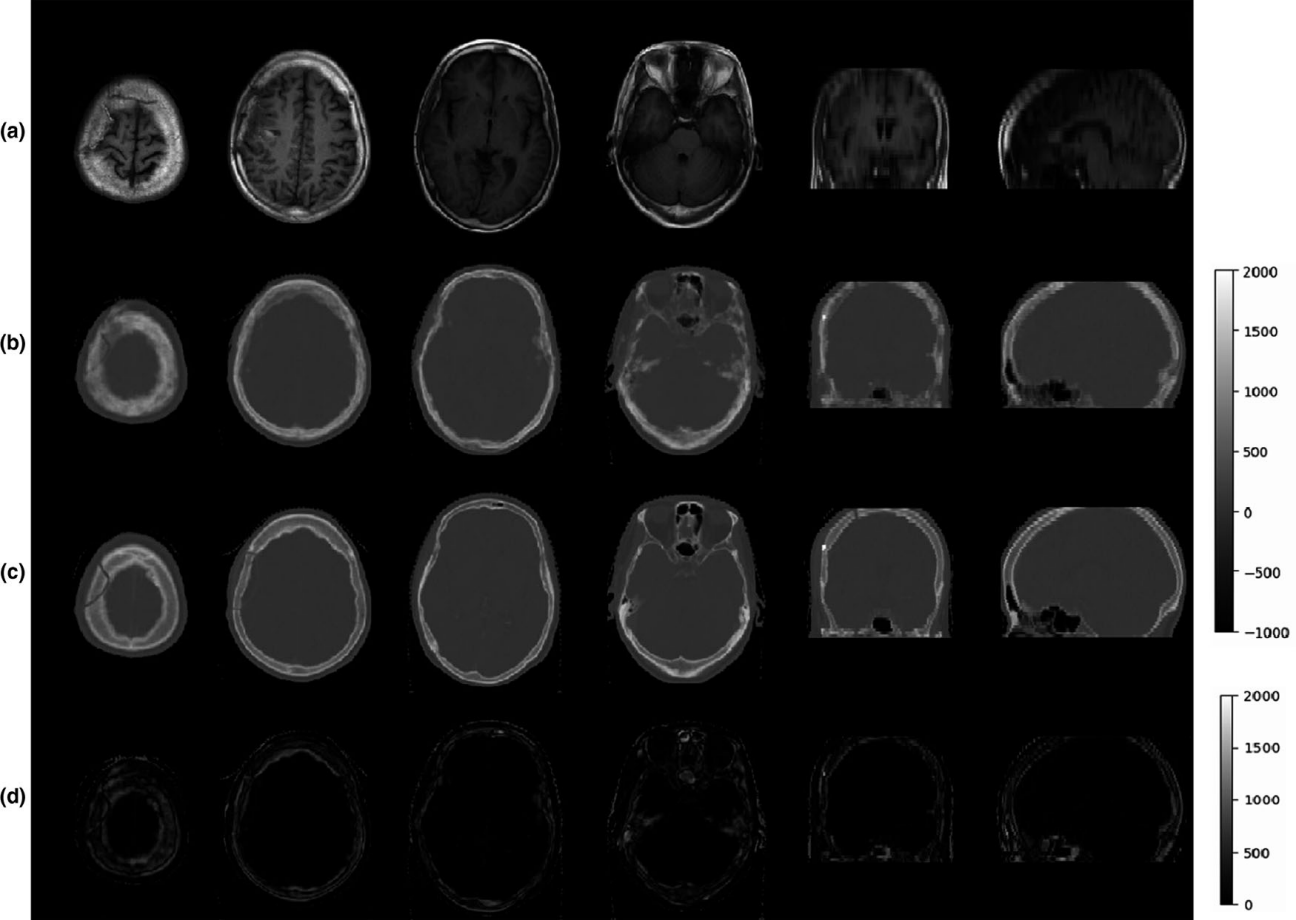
Comparison of (a) T1‐weighted TIRM Dark Fluid MRI, (b) synthetic computed tomography (CT), (c) planning CT and (d) difference map in transverse, sagittal, and coronal views for one example patient.
